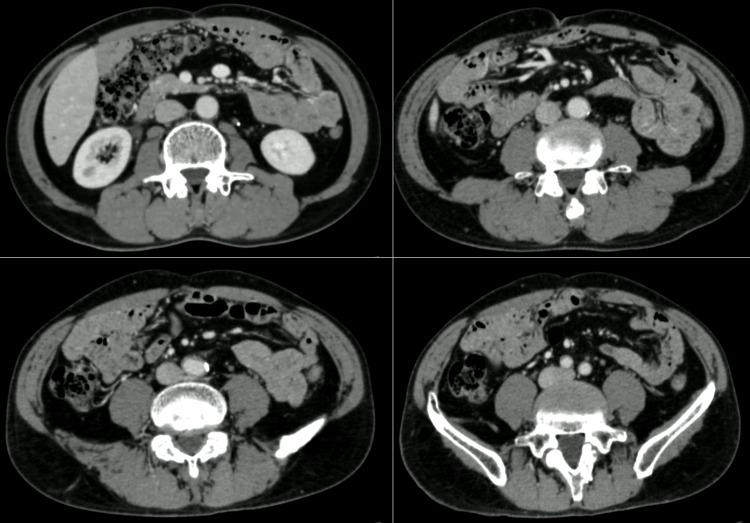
Contrast-enhanced CT at 8 years after operation There is no obvious local recurrence or distant metastasis.
